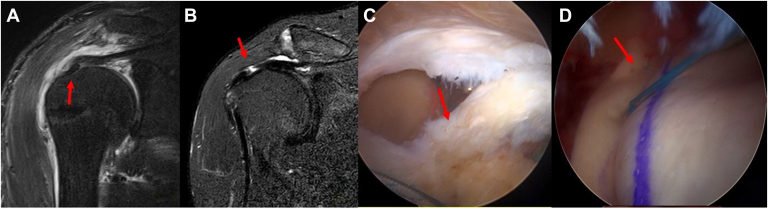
Re-revision rotator cuff repair of the partial articular side supraspinatus retear (PASR) lesion. (A) A revision rotator cuff repair with biceps tendon augmentation (*arrow*) without tear completion for PASR lesions was performed. (B) One year postoperatively, follow-up MRI demonstrated a full-thickness rotator cuff retear. (C) Arthroscopic evaluation confirmed a full-thickness rotator cuff tear measuring 1.5 × 1.5 cm. However, the previously augmented biceps tendon remained well incorporated into the rotator cuff footprint (*arrow*). (D) A rerevision bridging rotator cuff repair was subsequently performed using an acellular dermal matrix. *MRI*, magnetic resonance imaging.
